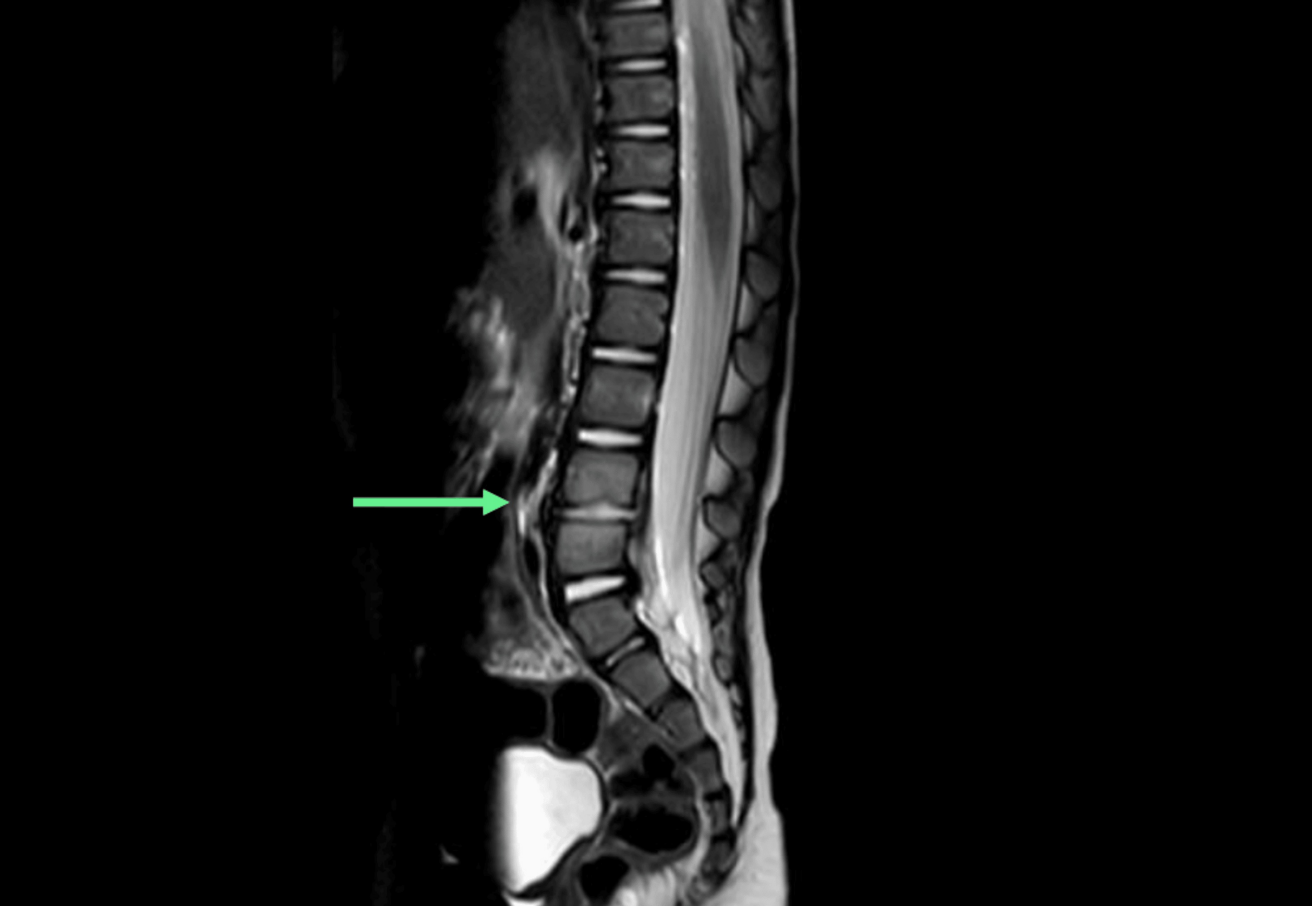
Sagittal view of an MRI of the lumbosacral spine. An arrow points to the disc between the L4 and L5 vertebrae. Fluid signal within the disc with minor loss of disc height and early end plate erosion of L4 and L5 vertebrae. Infective material/pus remains confined within the disc annulus, with no epidural hematoma or canal narrowing seen. MRI: magnetic resonance imaging
